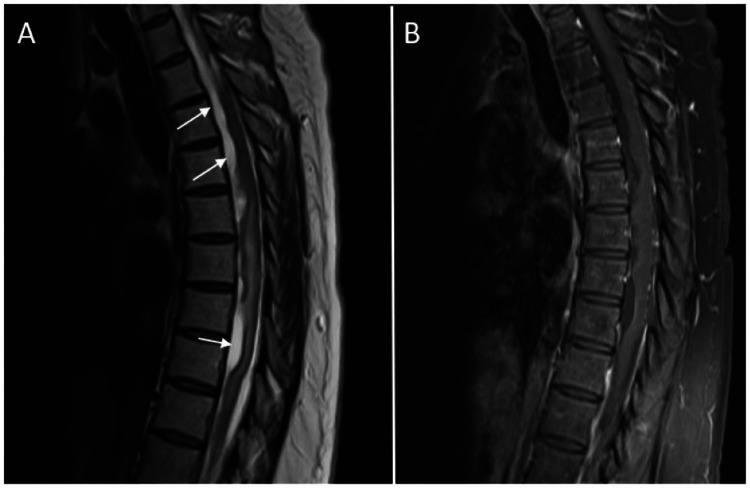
Postoperative sagittal MRI images from case two Sagittal MRI images of the patient's thoracic spine from case two at three months postoperatively showed arachnoid cysts. (A) T2 sequence showing the arachnoid cysts (white arrows); (B) Post-contrast T1 sequence showing no abnormal enhancement.
